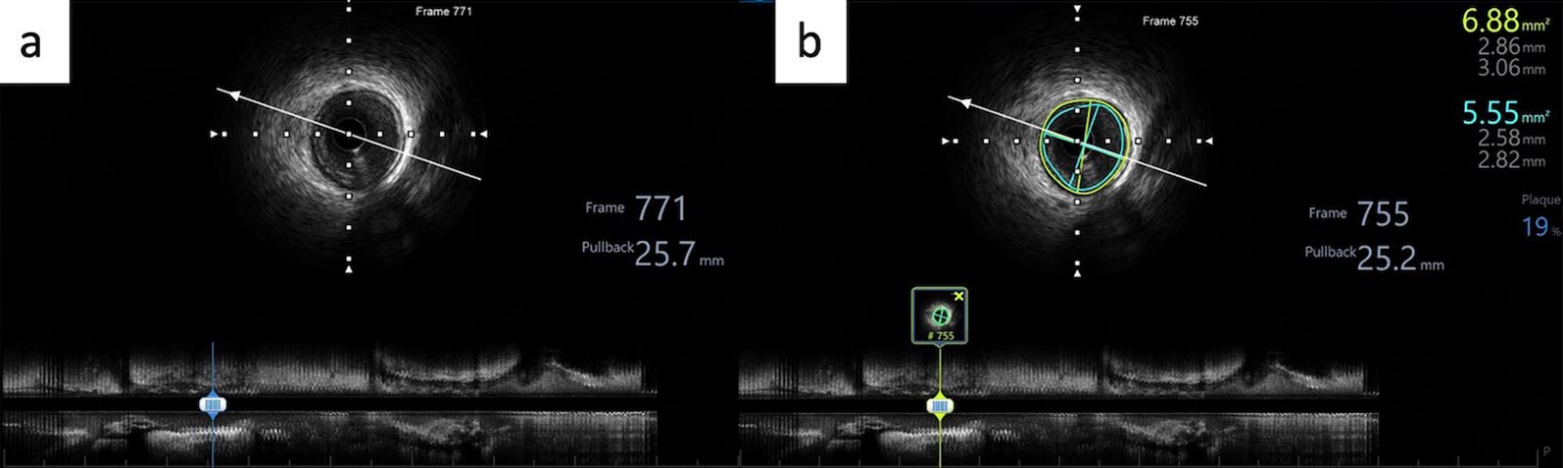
IVUS Image Acquisition and Measurement. A IVUS longitudinal view; B EEM CSA tracing and diameter measurement. CSA = cross-sectional area; EEM = external elastic membrane; IVUS = intravascular ultrasound
